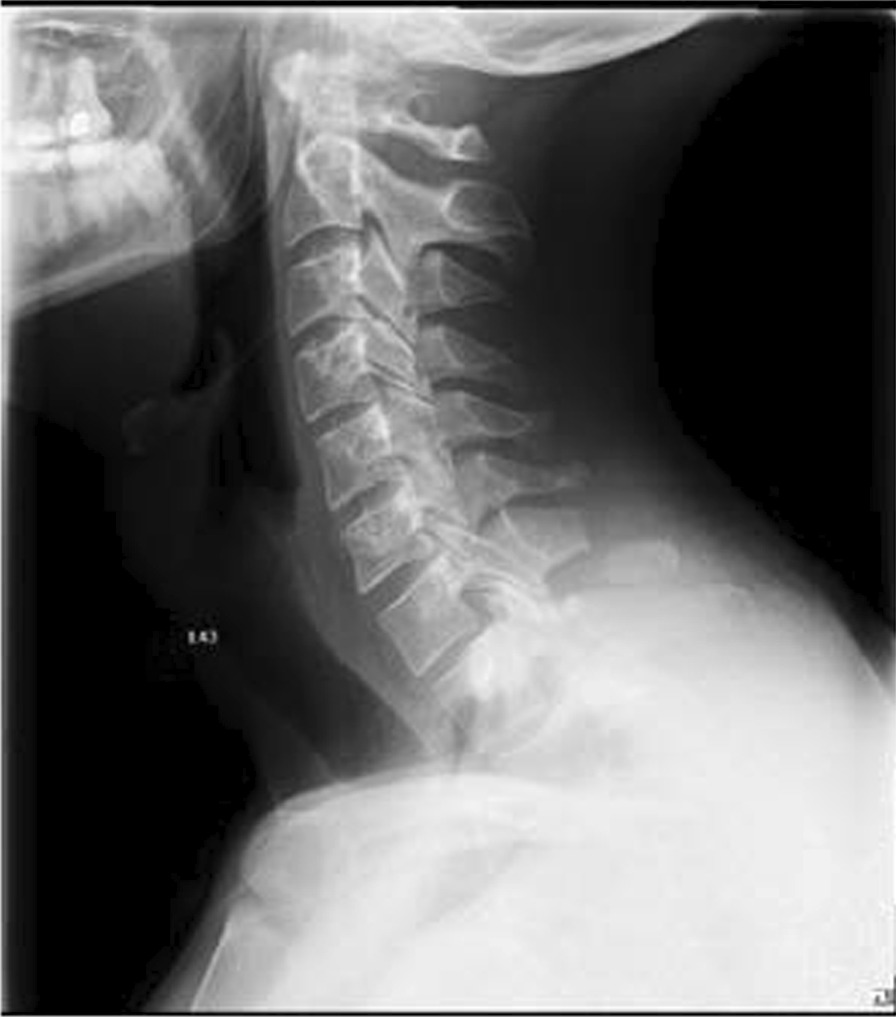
Lateral neck X-ray taken at admission. X-ray, computed tomography and magnetic resonance imaging taken from patient folder with the patient’s consent
